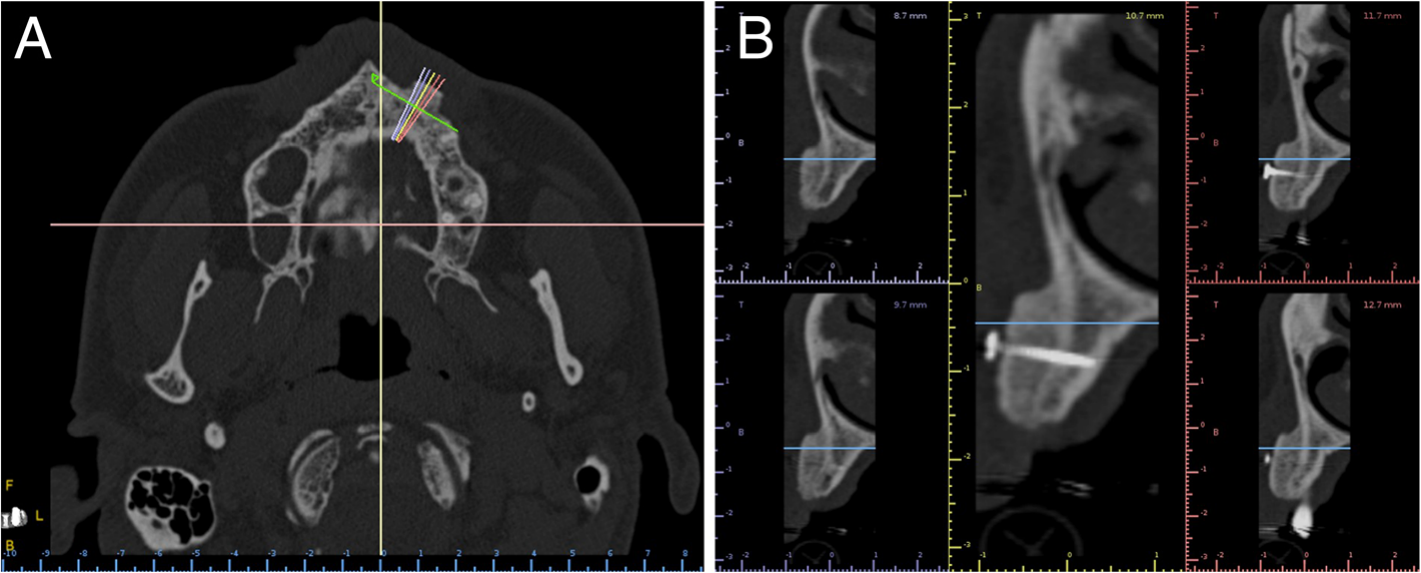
CBCT were obtain after 6 months to determine implant width and length, on axial (a) and cross-sectional (b) images
